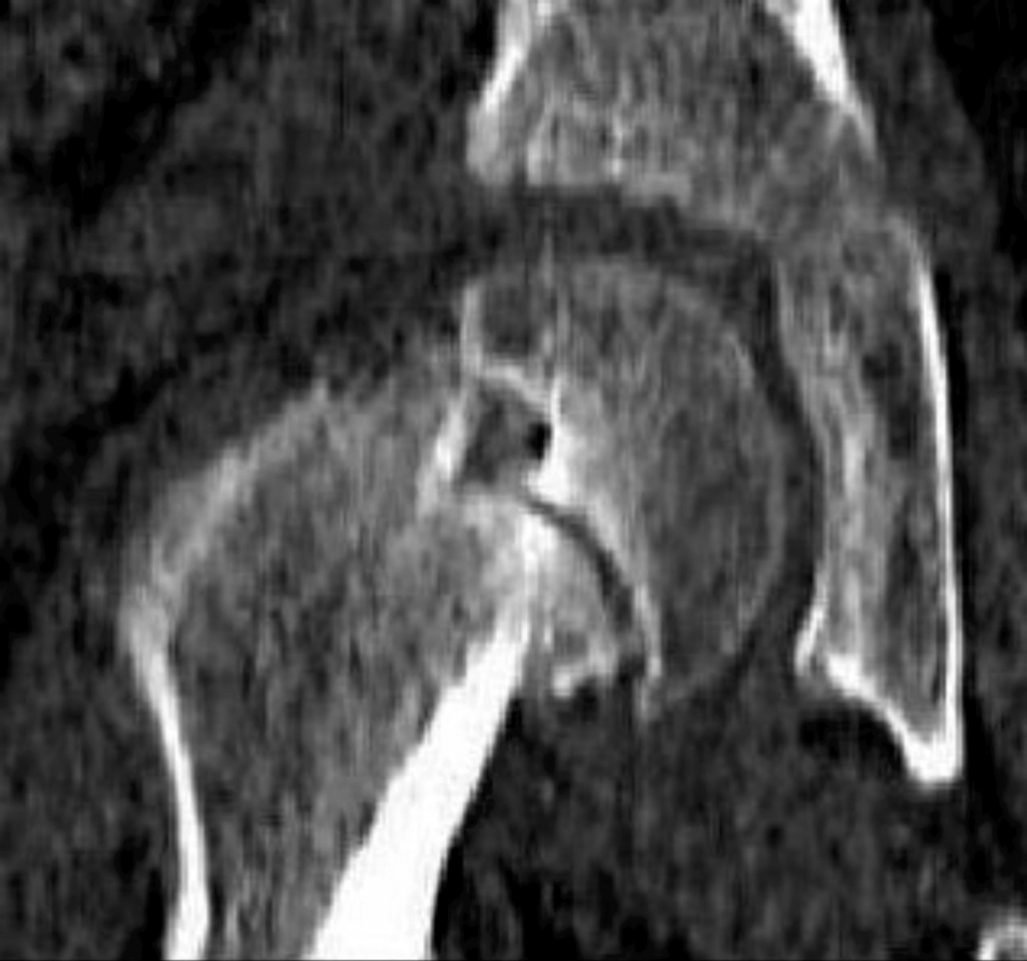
AP CT scanogram of the right hip of a 13-year-old boy, showing a well-developed epiphyseal tubercle that is clearly displacing out of the metaphyseal socket as an acute-on-chronic slip develops.
